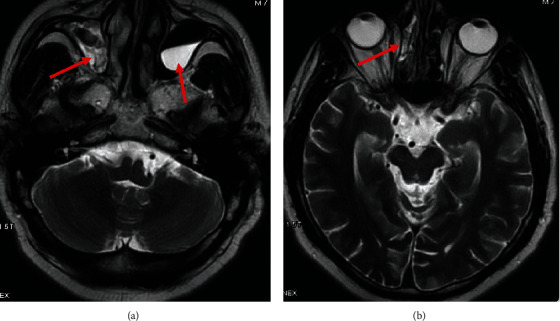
Axial T2-weighted images without contrast administration show (a) mucosal thickening and secretion in the right maxillary sinus and a retention cyst in the left maxillary sinus and (b) mucosal thickening and secretion in the right ethmoid sinus with a lesser degree in the left ethmoid sinus.
